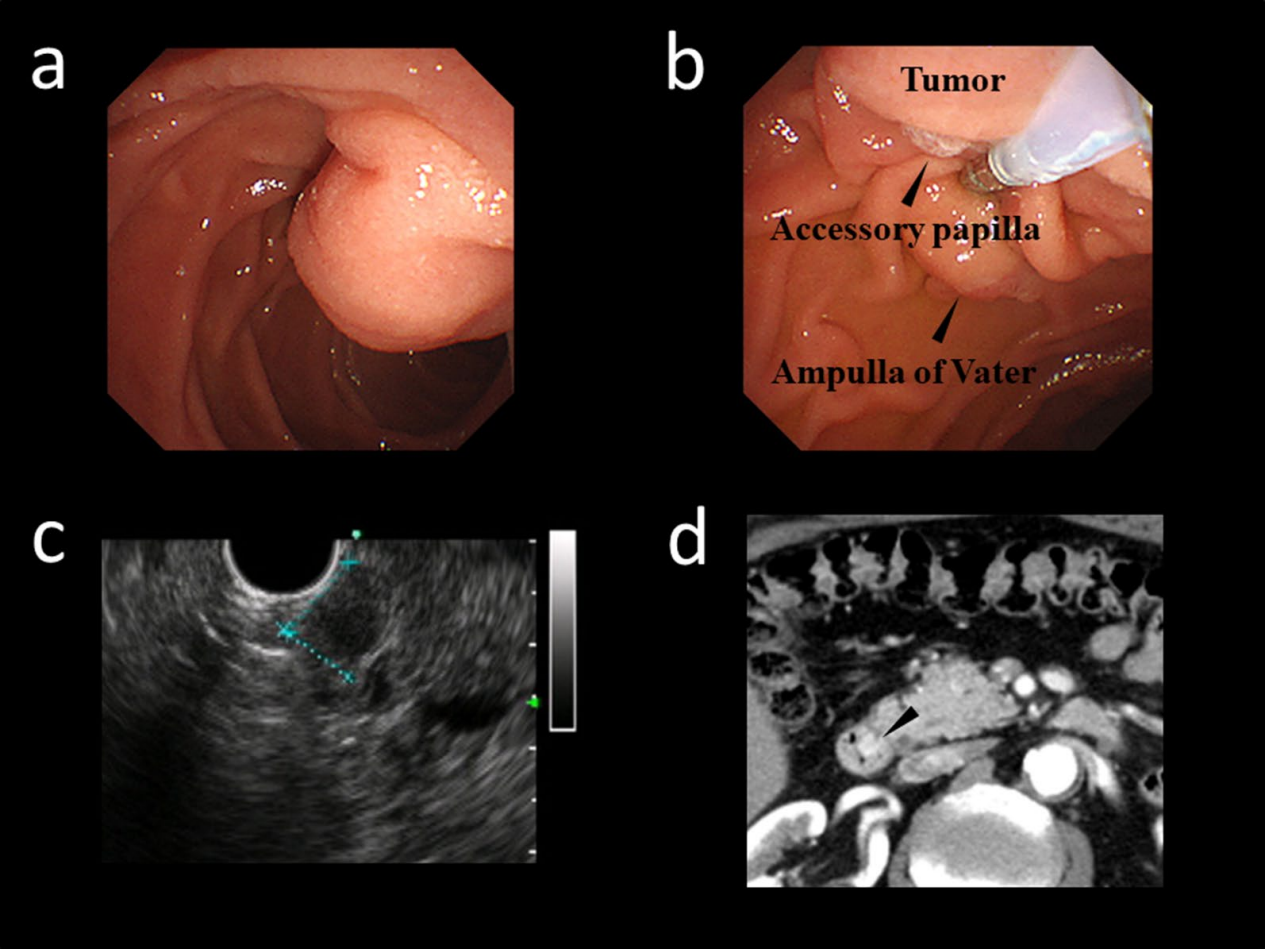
Endoscopic examination showed a 8-mm submucosal tumor at the accessory papilla in the second part of the duodenum (a). The relationship between the tumor, the accessory papilla and the ampulla of Vater is demonstrated (b). Endoscopic ultrasonography demonstrated hypoechoic mass in the submucosal layer (c). Computed tomography (CT) revealed the highly enhanced tumor in the early phase with no metastatic lesion including the lymph node and the liver (d)
